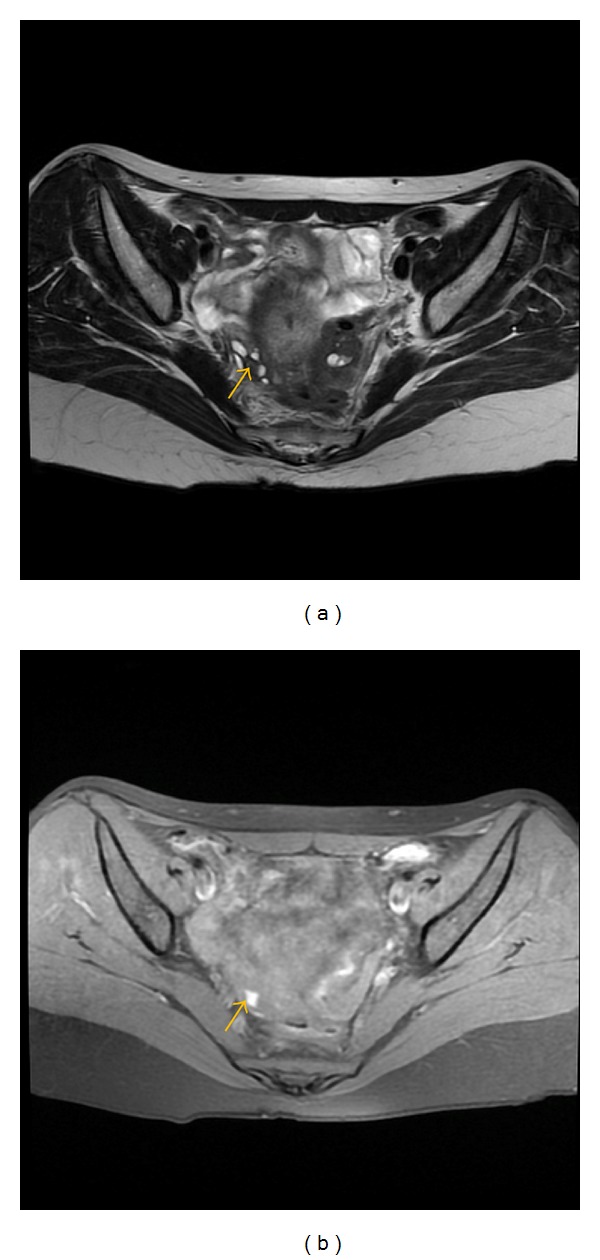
Axial (a) T2-weighted fast SE image (repetition time msec/echo time msec = 4640/102) shows typical hemorrhagic focus (arrow) as low signal intensity small lesion in the right ovary on T2-weighted image with corresponding high signal intensity on T1-weighted fast SE image (b) with fat suppresion (TR/TE = 600/7.6).
